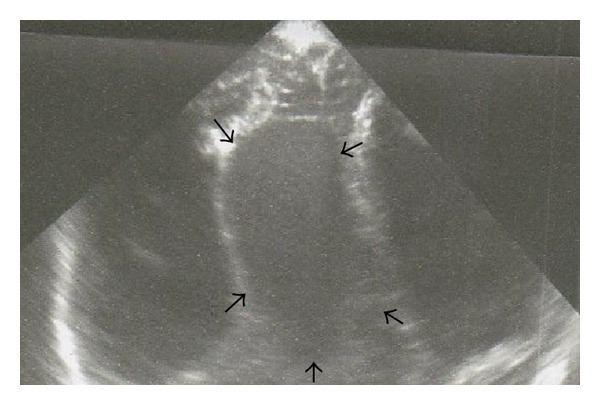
Ultrasound imaging of our case's aneurysm of vein of Galen.
